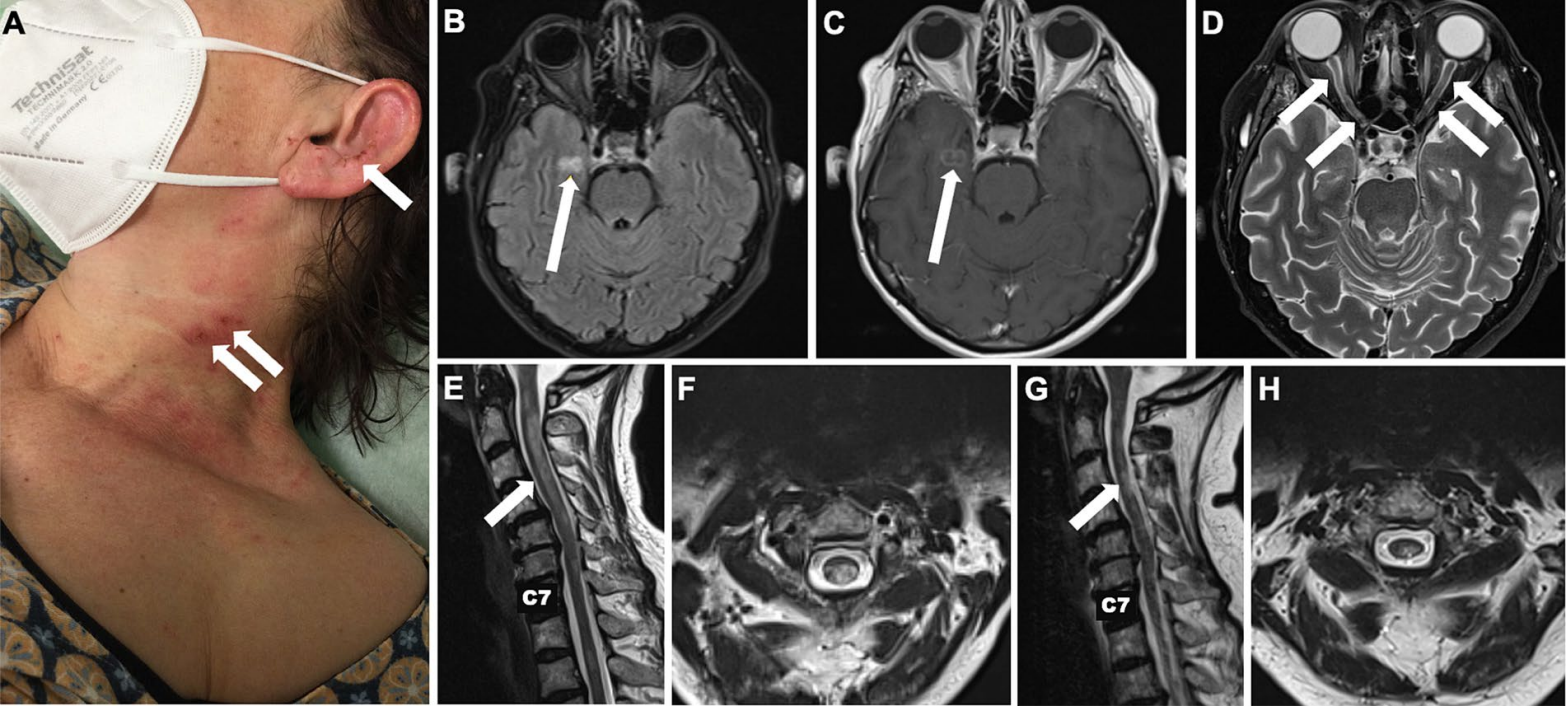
Skin changes and longitudinal MRI of brain and spinal cord. Localised painful rash with crusts on the neck and lateral face initially due to *repetitive scratching* in central neuropathic pain syndrome initially misdiagnosed as herpes zoster reactivation (A). Cerebral T2-weighted FLAIR MRI showing a new hyperintense parahippocampal lesion that evolved within 6 days from symptom onset (B) and presented gadolinium enhancement (C). T2 hyperintense lesions in both optic nerves consistent with optic neuritis (D). Hyperintense spinal cord lesions compatible with cervical longitudinally extensive transverse myelitis reaching from the atlas to the 6th vertebra upon admission to our tertiary care centre (E,F) and after 6 months (G,H).
